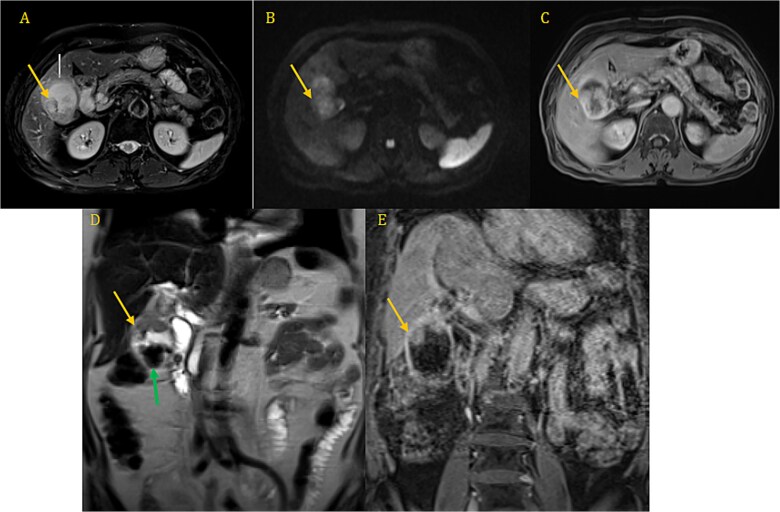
CP-MRI demonstrates markedly dilated gallbladder with cholelithiasis (green arrow) and wall thickening involving the superior portion and the infindubulum (yellow arrow) in iso-signal on T2 (A, D), restrictive on DWI (B), with a late enhacement after injection (C, E).
